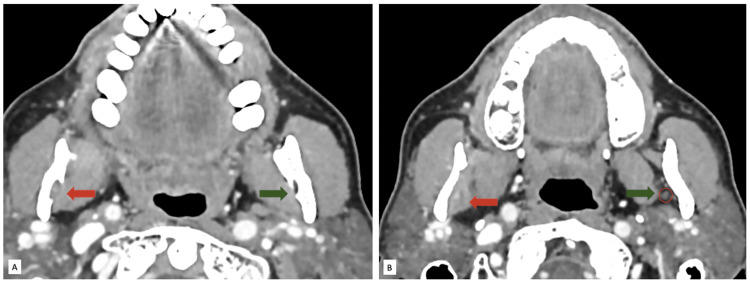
Contrast-enhanced CT neck images in the axial plane at the level of the ramus of the mandible. (A) Widened right mandibular foramen (red arrow); normal mandibular foramen on the left (green arrow). (B) Soft tissue surrounding (red arrow) the right inferior alveolar nerve. Normal inferior alveolar nerve on the left side (red circle) surrounded by fat (green arrow).
